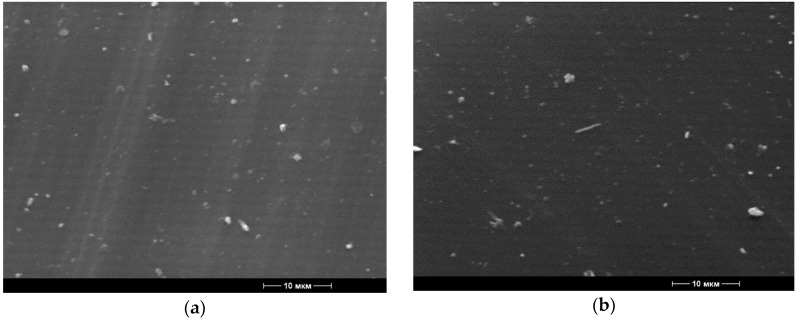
Microphotographs of the surface structure of the materials (5000× magnification) based on polyethylene with 2.0% content of birch bark extract, received with the use of ultrasonic treatment (a) and without the use of ultrasonic treatment (b).
